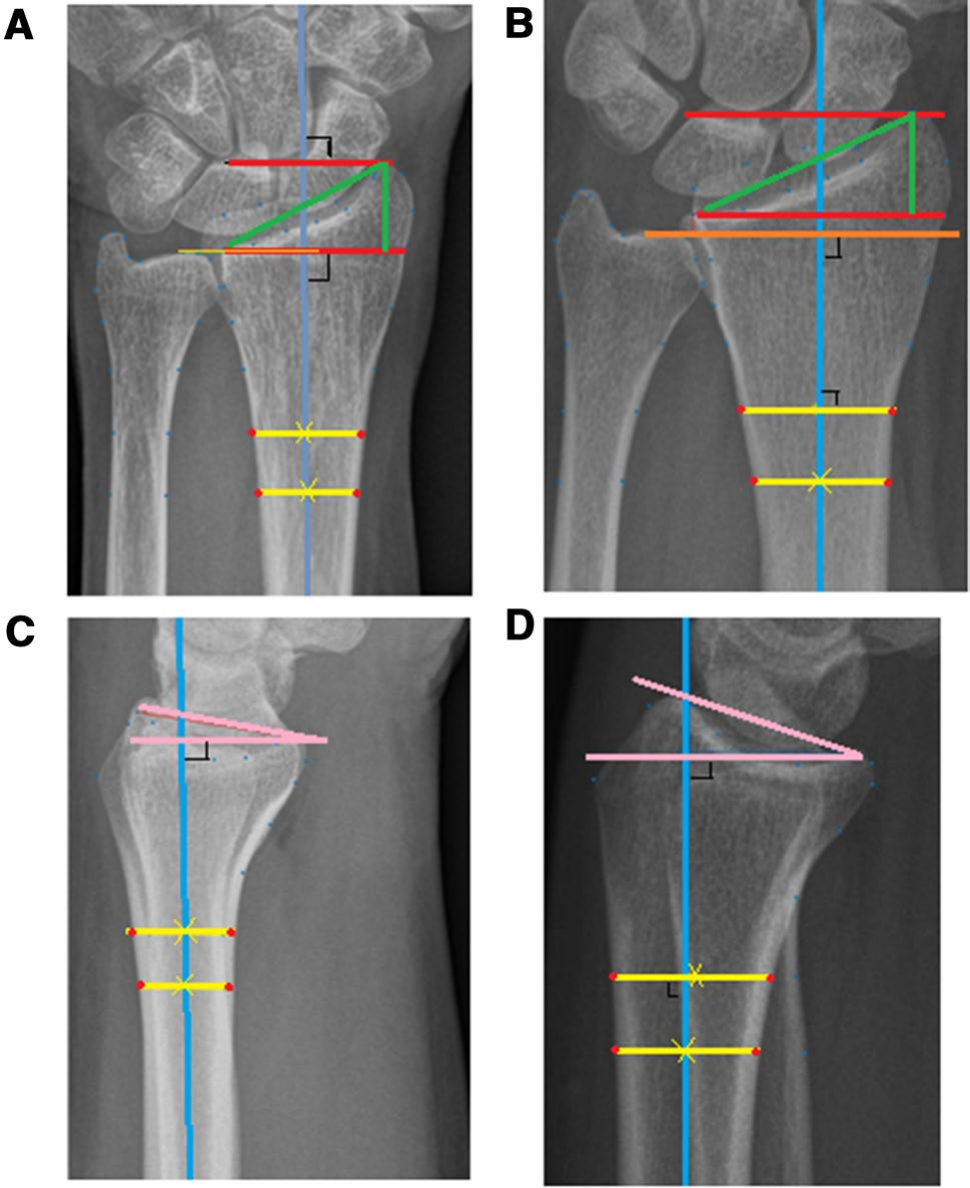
Linear measurements. A, C Perpendicular line to the long axis of the radius is drawn through the middle of the two yellow lines or B, D through the middle of the proximal yellow line and perpendicular to the distal yellow line. A, B Posteroanterior wrist radiographs with measurements of (1) Ulnar variance: the orange line, which is touching the distal cortical rim of the ulna, is proximal (minus) or distal (plus) to the red line which is touching the distal ulnar aspect of the radius. (2) Relative radial height: The length of the proximal red line divided by the length of the vertical green line which is touching the tip of the radial styloid. (3) Radial inclination: The angle between green line from the distal ulnar aspect of the radius to the tip of the radial styloid and the proximal red line. C, D Lateral wrist radiographs with measurements of volar tilt: The angle between the pink lines which is touching the most distal points of the volar and dorsal ridge of the radius
